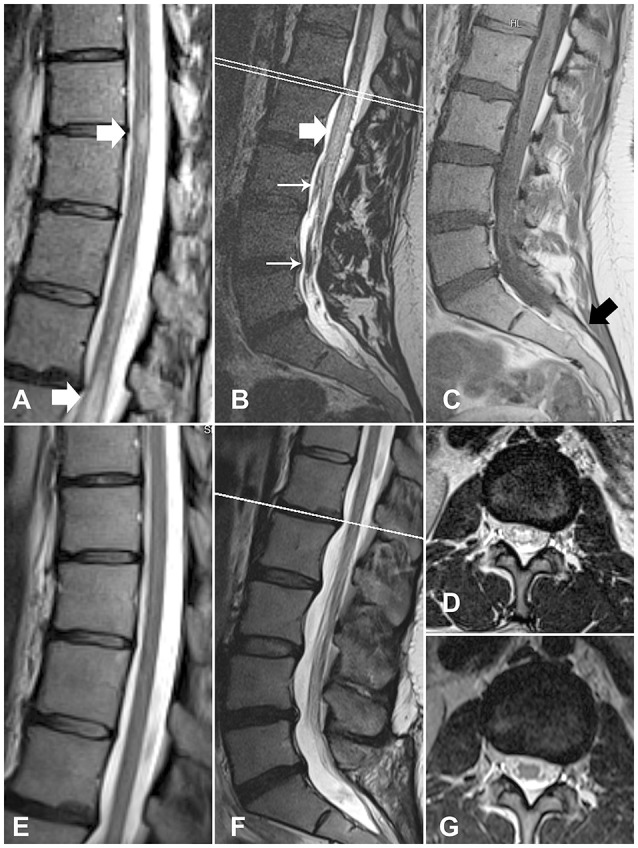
MR images of the spinal cord of case 1, before and after treatment. (A) Myelopathic changes in the thoracic spinal cord (white arrows) (B) Myelopathic changes in the conus medullaris (thick arrow) and thickened torturous vessels (thin arrows). (C)T1WI sequence shows a filum terminale lipoma (black arrow). (D) A transverse section at the L1/L2 level shows myelopathic changes. (E–G) Follow-up images demonstrate the resolution of myelopathic changes and the disappearance of the thickened veins.
